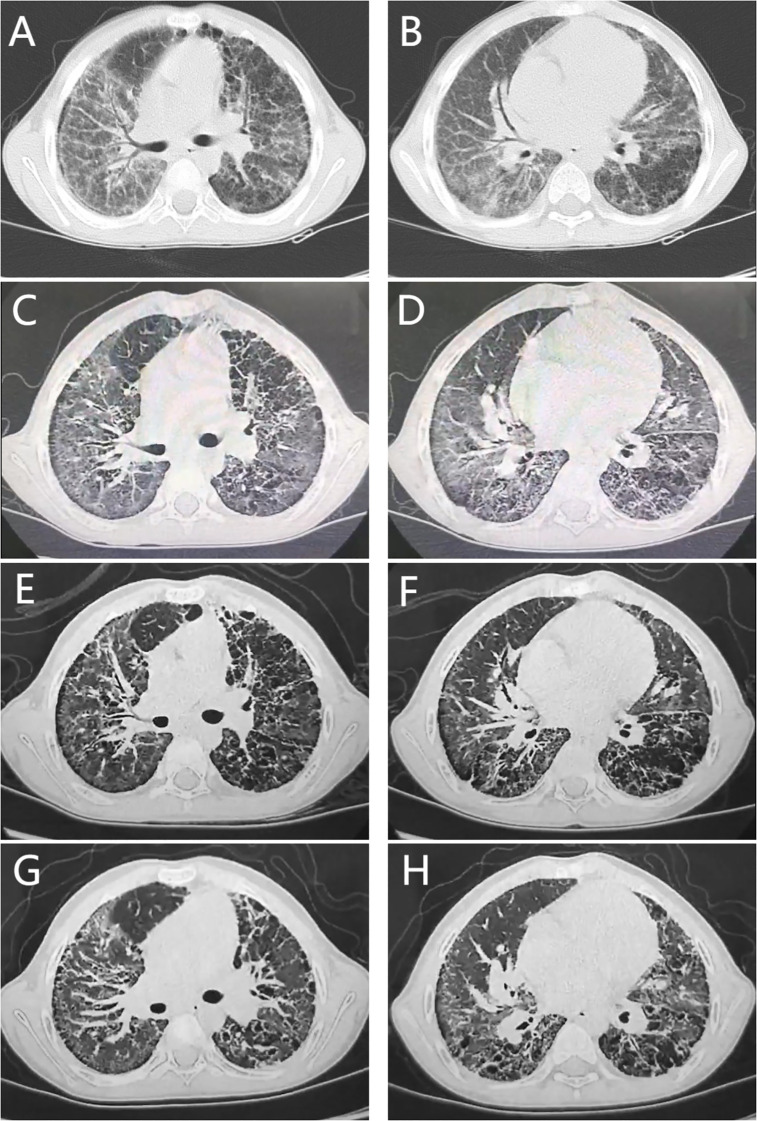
Chest computed tomography findings. (A, B), the CT scan before tofacitinib. (C, D), the CT scan 1 year after tofacitinib. (E, F), the CT scan 2 months after ruxolitinib. (G, H), the CT scan 1 year after ruxolitinib. All CT scans showed diffuse reticular shadows in the lungs, multiple subpleural microcystic lesions, and thickening of the interlobular septa. Neither tofacitinib nor ruxolitinib treatment could prevent the increase in the number and size of cystic shadows. (E–H) showed honeycombing changes and worsening emphysema.
